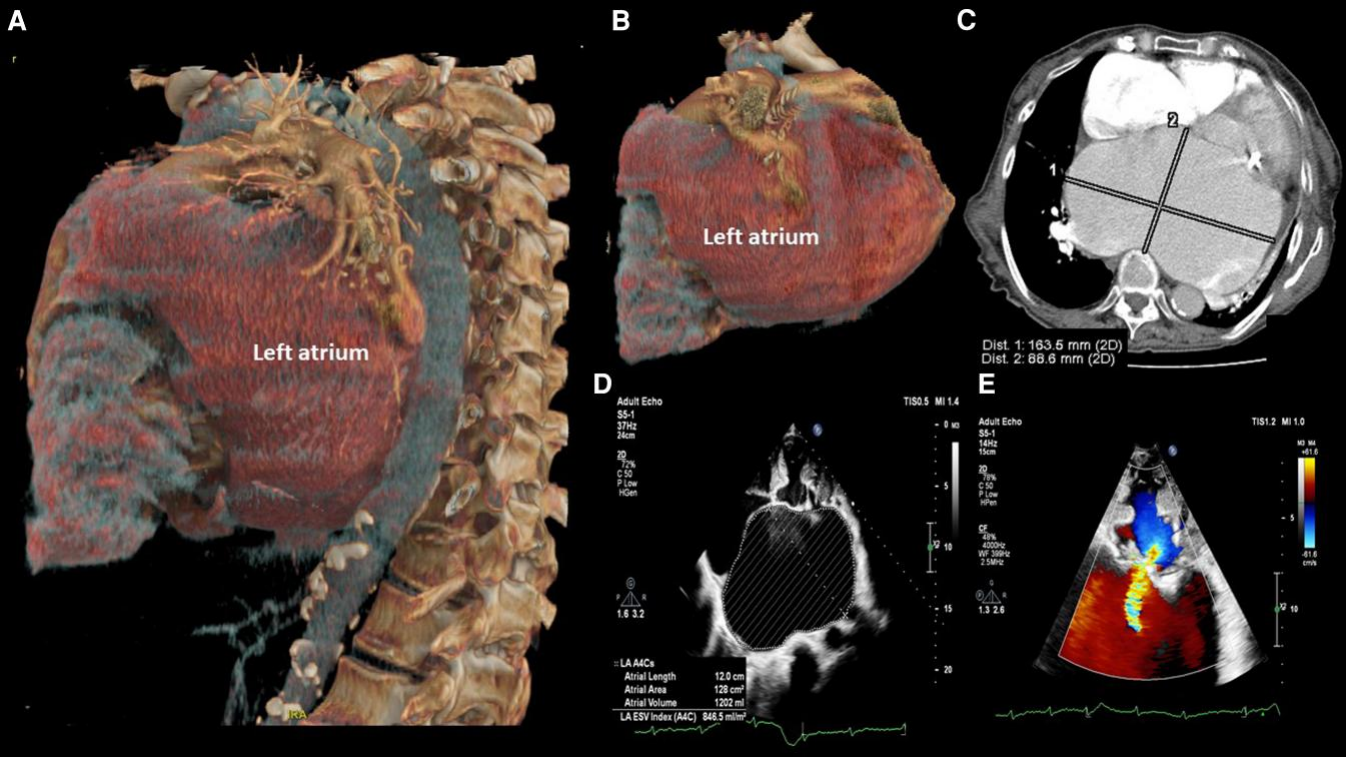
Multimodal imaging of a giant left atrium. *(A)* Computed tomography 3D reconstruction (left lateral view) highlighting the enlarged left atrium in contact with the spine, causing displacement of the oesophagus and descending aorta. Pulmonary vessels, left atrial appendage, left ventricle, and thoracic aorta are also visible. *(B)* Computed Tomography 3D reconstruction (left lateral view) highlighting the enlarged left atrium, shown without the spine, demonstrating deformation of the giant left atrium due to compression by the vertebral column. *(C)* Axial computed tomography image at the level of the mitral valve showing mitral annular calcification, predominantly involving the posterior mitral leaflet with left atrial dimensions of 163.5/88.6 mm. *(D)* Apical four-chamber view demonstrating a markedly increased left atrial volume index of 846.5 ml/m^2^. *(E)* Apical four-chamber transthoracic echocardiographic view showing thickened mitral valve leaflets consistent with degenerative changes, along with a central mitral regurgitation jet. Concentric left ventricular hypertrophy is also noted.
